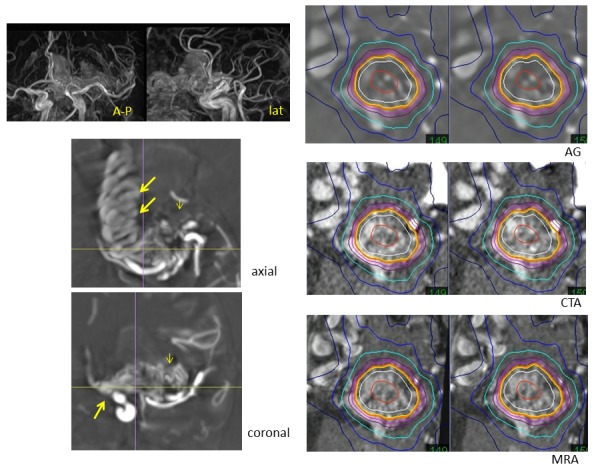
A brain arteriovenous malformation. MR angiography showing a large AVM in the left frontal lobe (upper left). The 3-D reconstruction from axial images of rotation angiography (lower left) showing the nidus (small arrow) and massive draining veins (large arrows) in the frontal base. Dose plan targeting of the nidus, excluding massive drainers, on axial images of the angiogram (AG) fused to those of CT angiogram (CTA) and enhanced MR angiogram (MRA).
